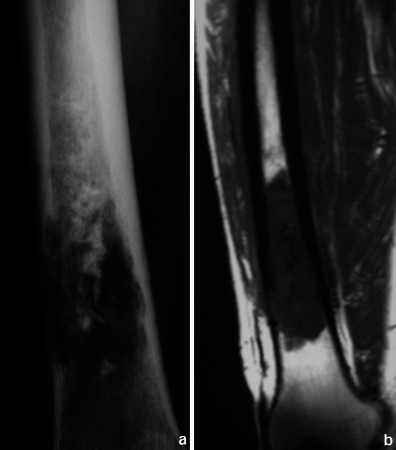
This patient had chronic lower thigh pain. The radiograph (a) showed an area of bone destruction with central calcification. Coronal T1WI clearly showed a hypointense lesion extending beyond the disrupted cortical tissue. This lesion was a chondrosarcoma
